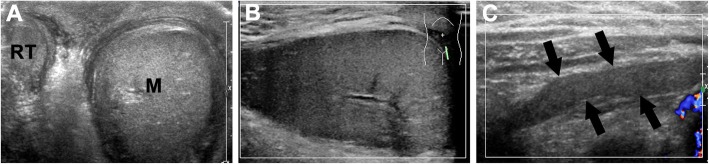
Ultrasound manifestation: a A mass (M) in the left scrotum with echo similar to that in the right testis (RT). b No blood flow signal in the mass. c The connection band (arrows). One end of the band was connected to the left scrotum mass, and the other end was extended to the abdominal cavity
